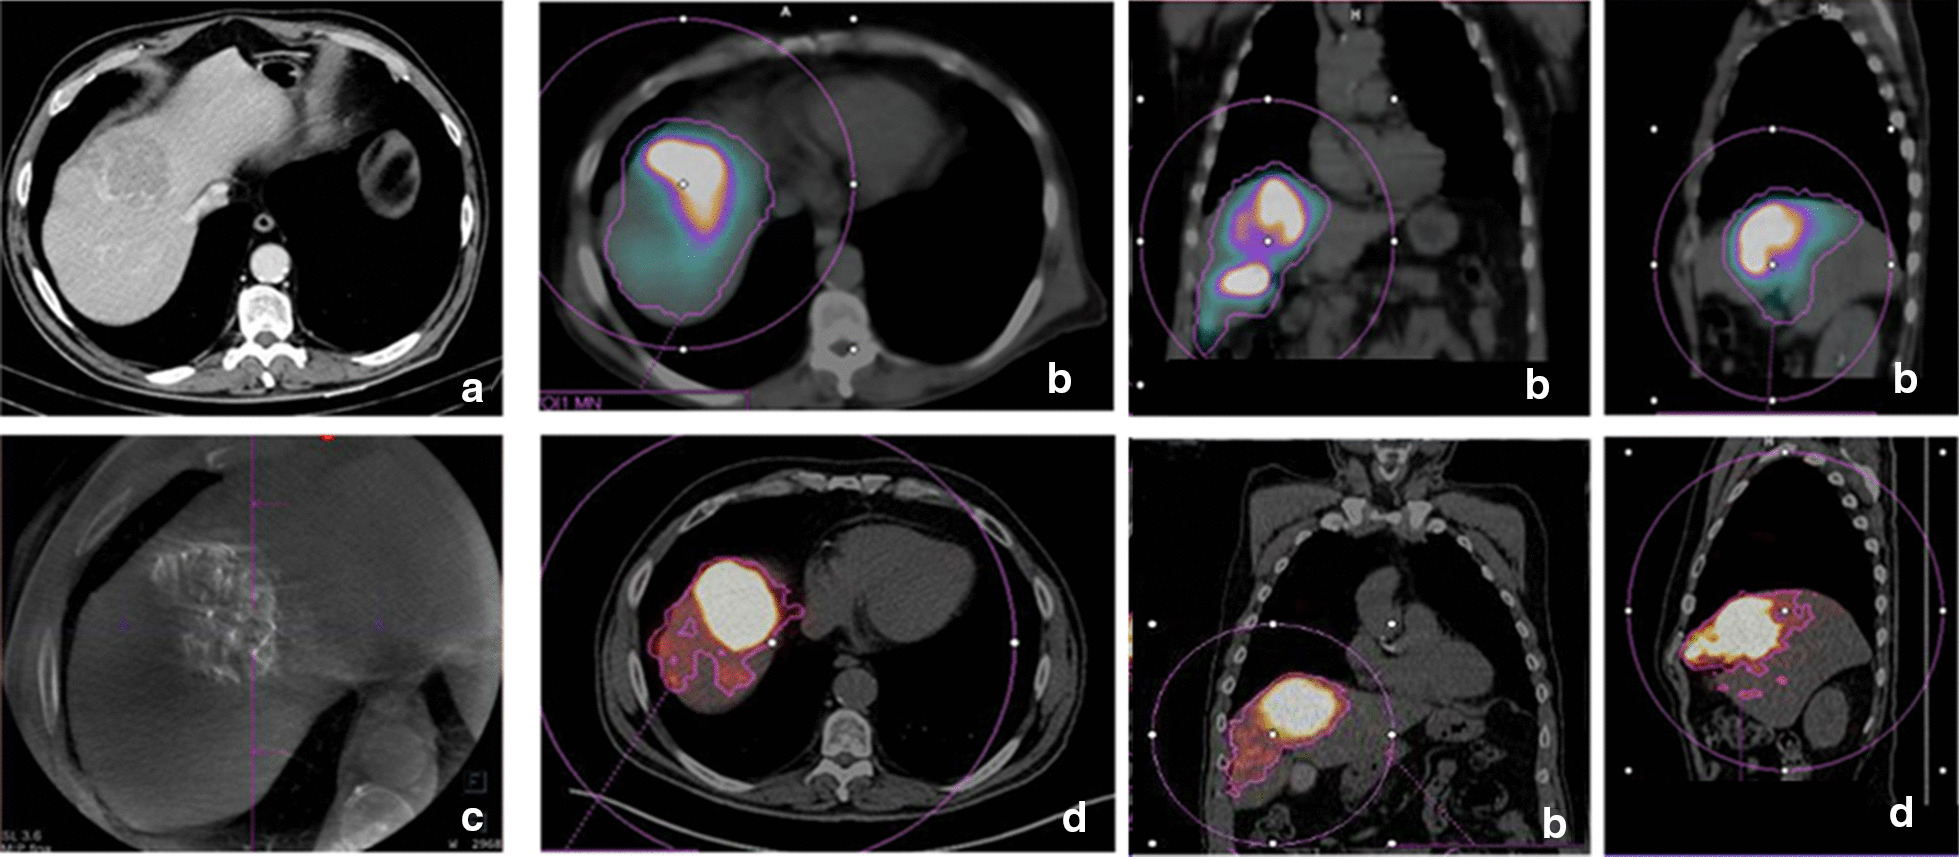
a Contrast-enhanced computed tomography (CECT) image in a patient with HCC located between segments IV and VIII. b Volumetric assessment of the target volume in SPECT/CT fusion images after the injection of ^99m^Tc-MAA through IV and VIII segments arteries. The volume was obtained using a “volume of interest and isocontour” tool, drawn in purple. c C-arm cone-beam CT (CBCT) showing contrast uptake in the tumoral lesion with no perfusion in non-tumoral parenchyma. d Volumetric assessment of the final target volume in the ^90^Y PET/CT fusion images
